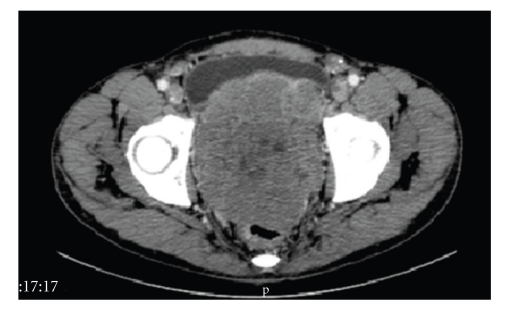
Pelvis CT showing a large pelvic mass.
